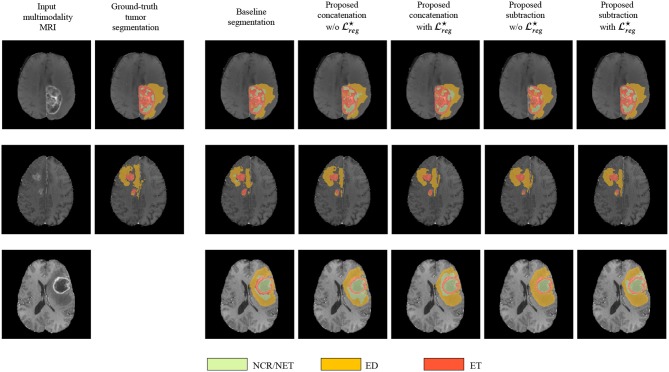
The segmentation maps produced by the different evaluated methods displayed on post-contrast Gadolinium T1-weighted modalities. We present the provided segmentation maps both on our test dataset and on the BraTS 2018 validation dataset. NCR/NET, necrotic core; ET, GD-enhancing tumor; ED, peritumoral edema.
